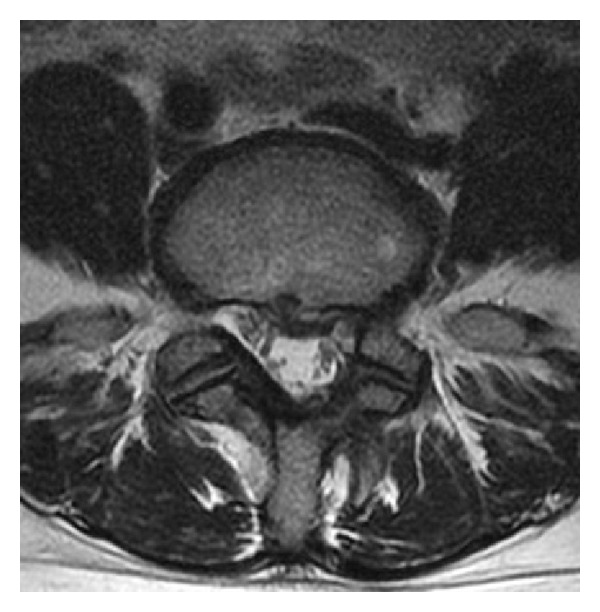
Preoperative magnetic resonance image showing a small recurrent herniated nucleus pulposus at L4-5 compressing the left L5 nerve.
